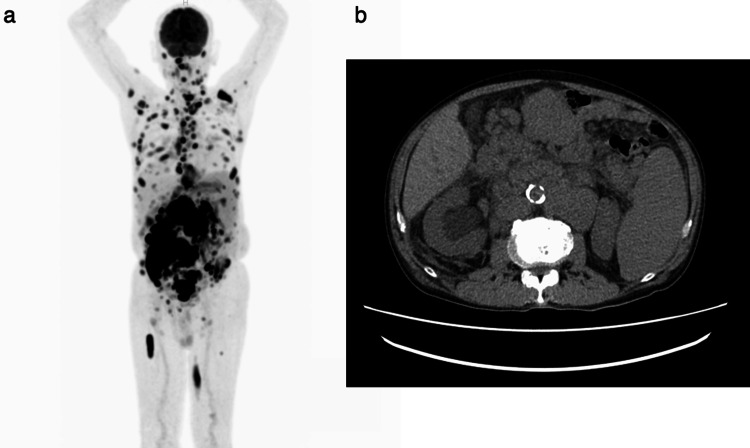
Positron emission tomography (a) and abdomen computed tomography (b). High 18F-fluorodeoxyglucose uptake in many swollen lymph nodes and bulky tumors of the abdomen was observed. Bulky abdominal tumors invaded the right ureter and induced hydronephrosis. Splenomegaly was also noted.
